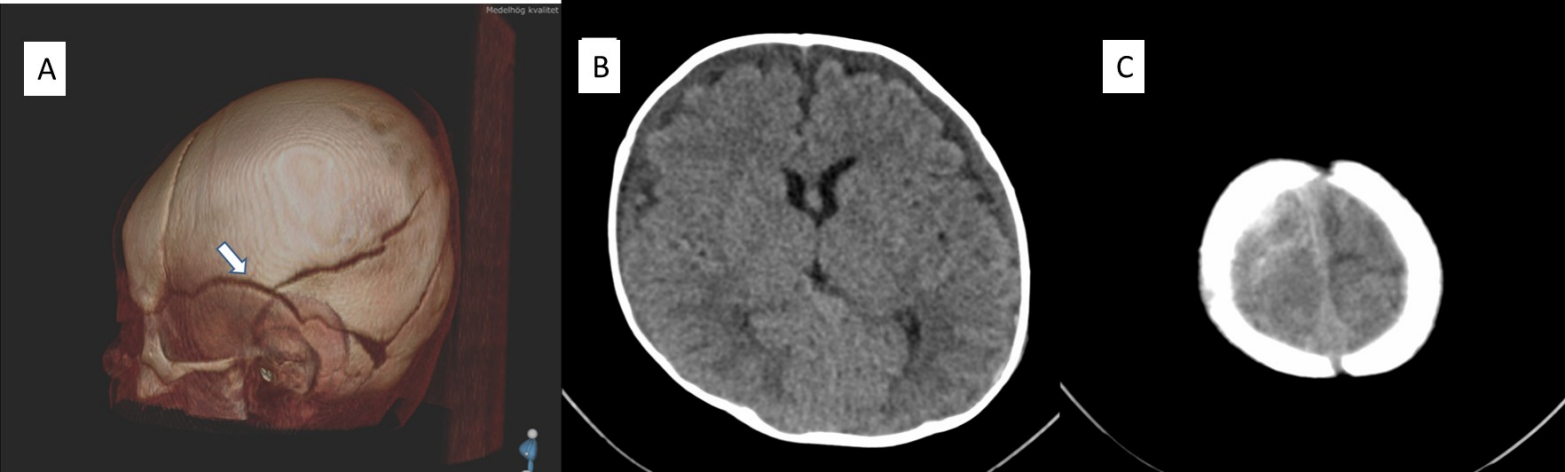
CT images of case 9. There is a temporoparietal fracture of uncertain age on the left side (arrow in A, 3D reconstruction with volume rendering technique). There are bilateral subdural fluid collections compatible with chronic subdural hematomas (B). There is a small amount of fresh subarachnoid blood bilaterally (C).
